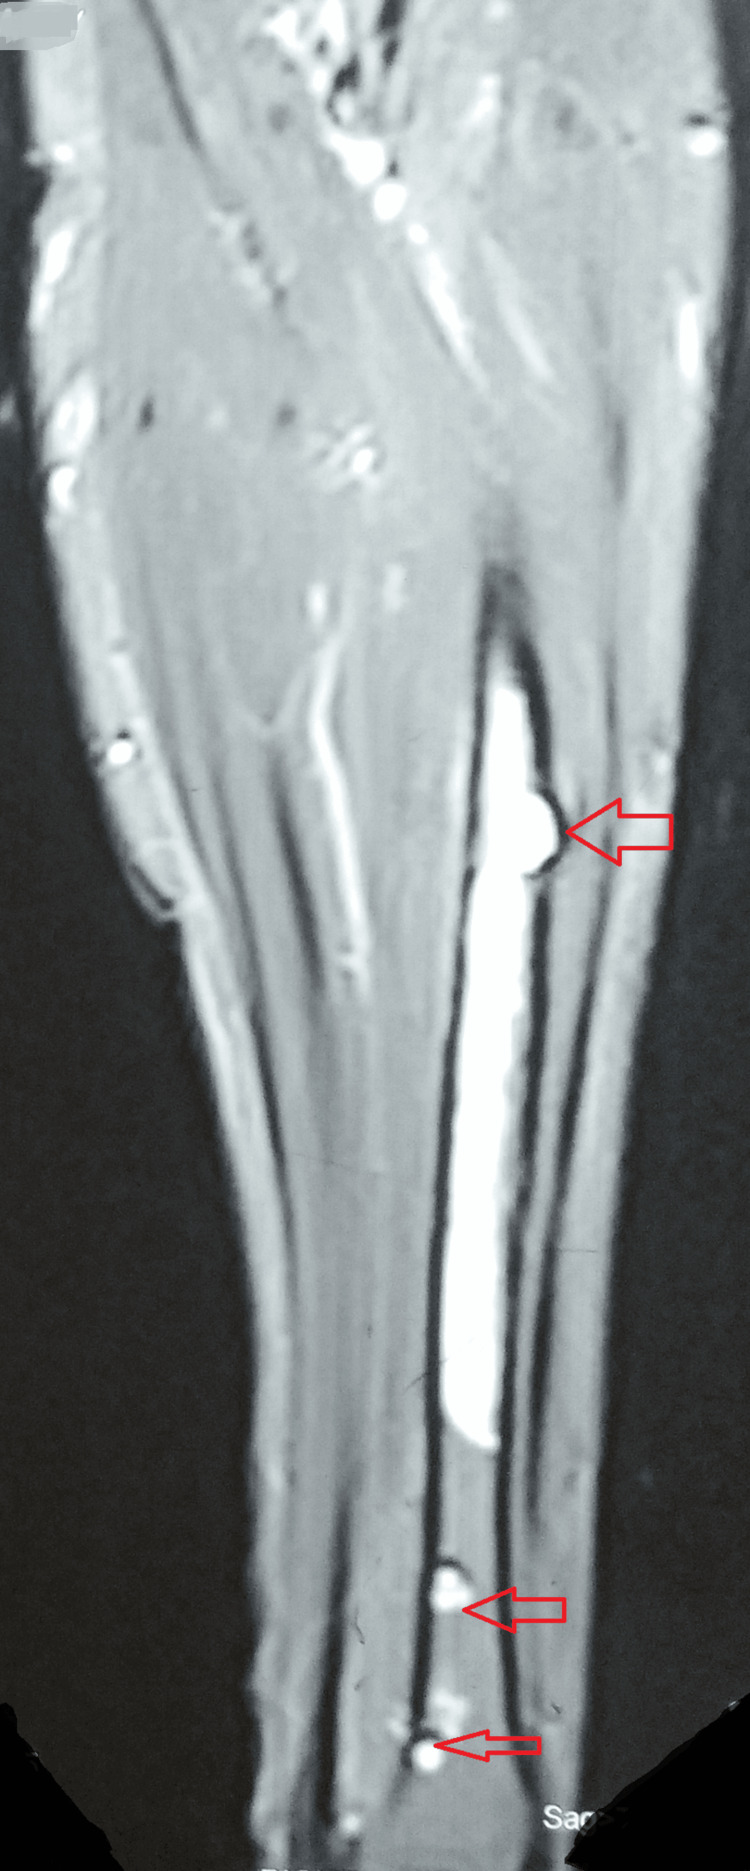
MRI of the left forearm Arrows indicate abnormal hyperintense marrow signals in the mid-shaft of the radius, approximately 11 cm in length. Similar small foci are observed in the distal shaft of the radius.
